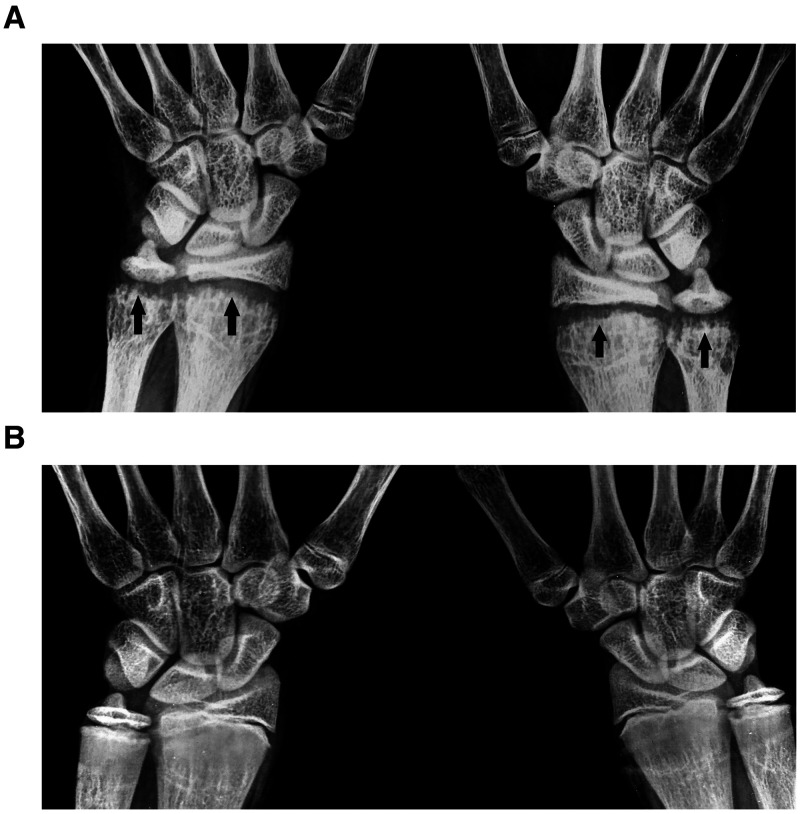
Radiographs of the wrists of the patient with severe rickets. (A) Frayed appearance of the distal metaphyses of radius and ulna at presentation (arrows). (B) Normalization of the changes, 6 months after treatment.
